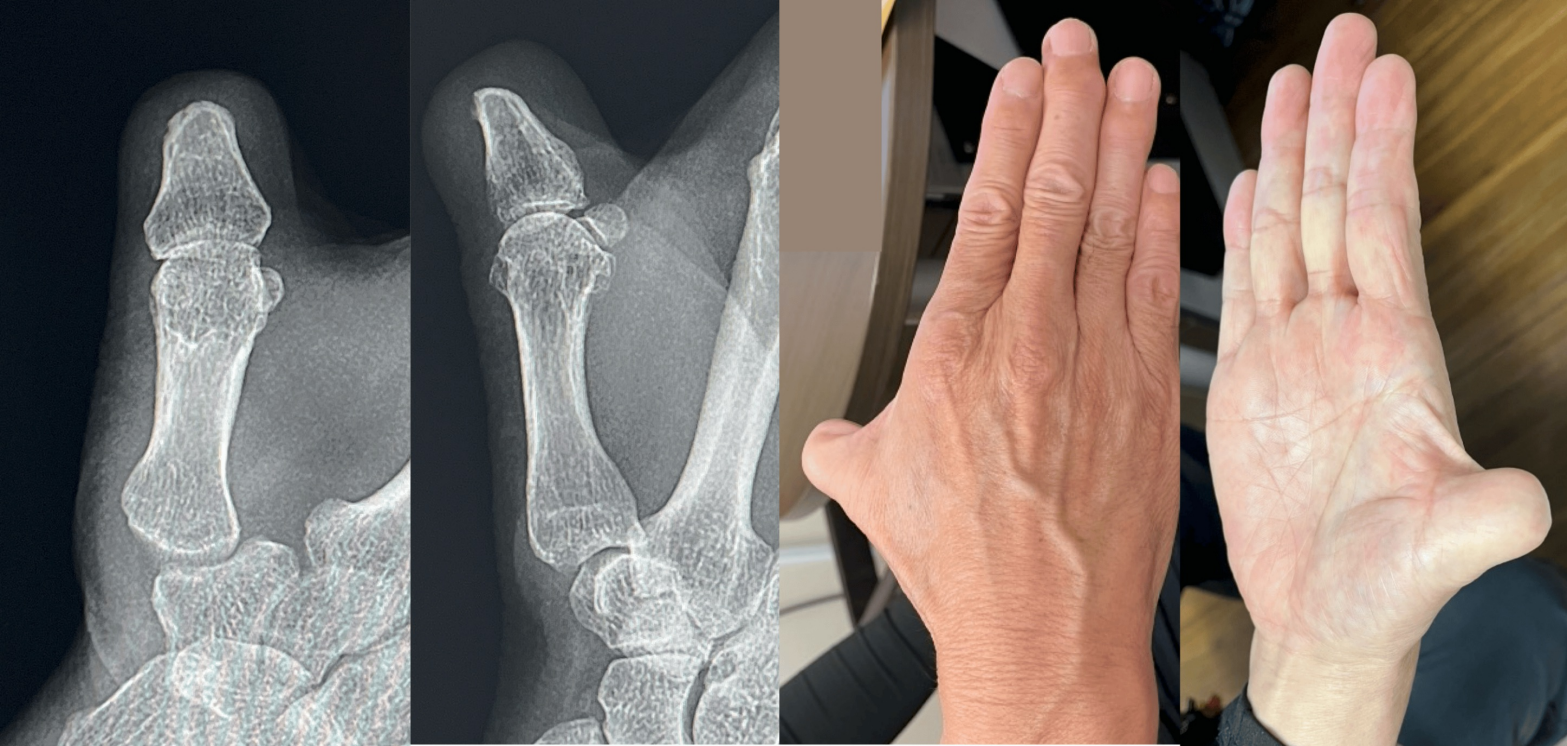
Postoperative radiographs and appearance at four years after the surgery.
